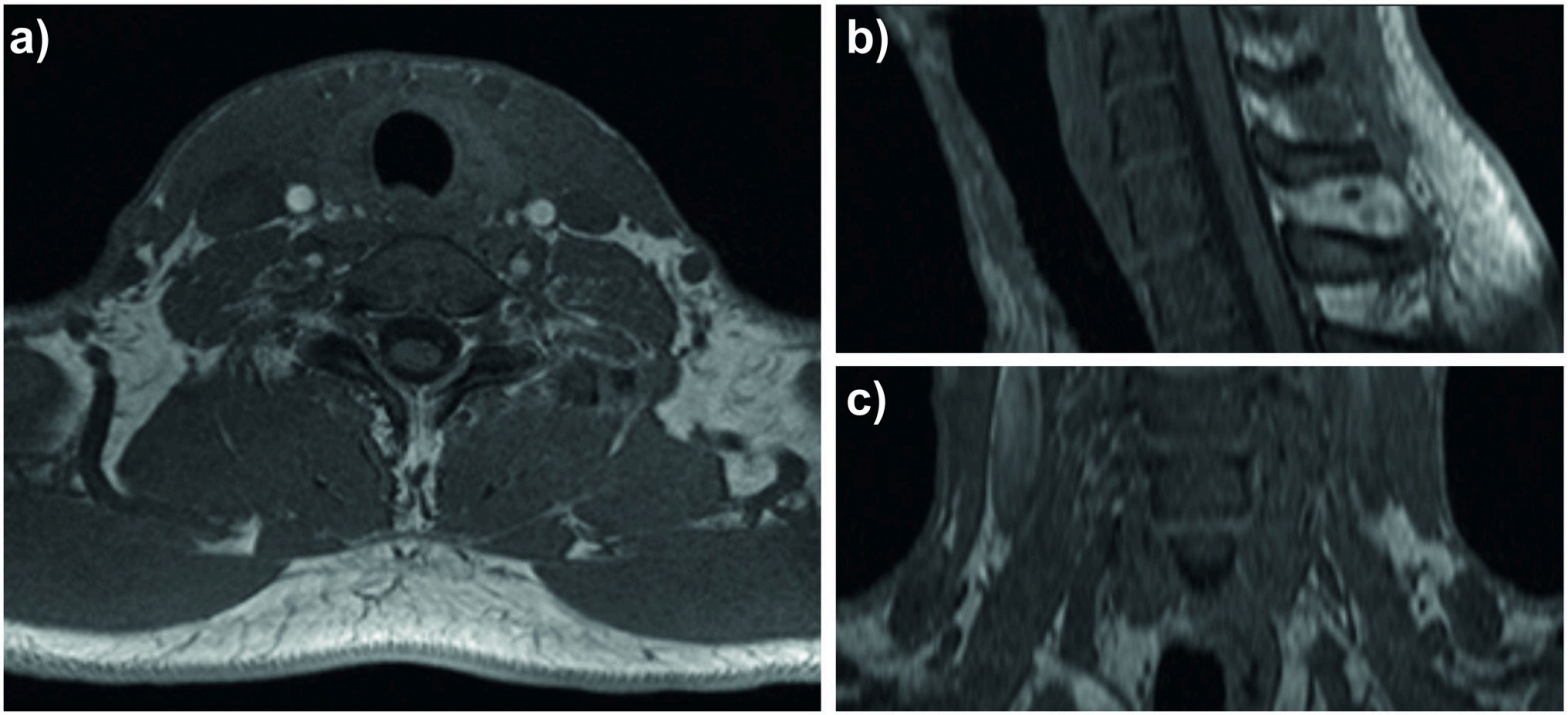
T1 VIBE MRI of the neck region for an exemplary volunteer, a) axial view, b) sagittal view, c) coronal view.
